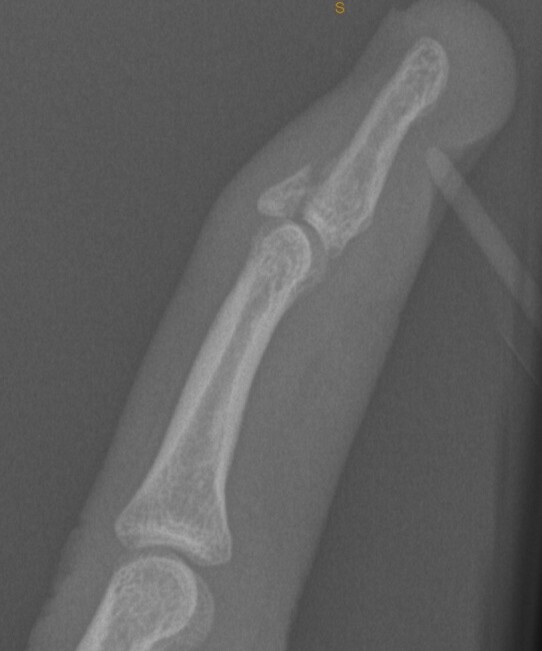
Lateral x-ray of ring finger: large dorsal avulsion fragment at insertion of Extensor digitorum longus (EDL) following catching injury
